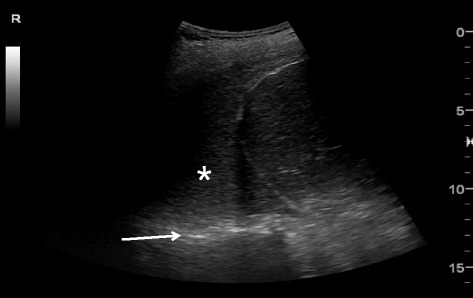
Lung consolidation. When the lung is consolidated (*asterisk*), it has a tissue-like appearance. The consolidation also allows penetration of the ultrasound beam, revealing the vertebral stripe (*arrow*)
